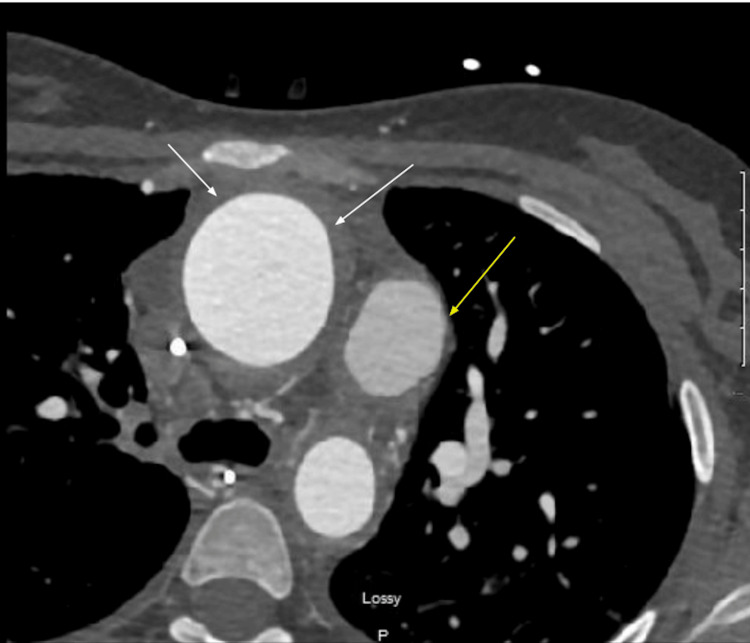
CT angiogram of the chest showing aortic wall thickening The white arrows show the ascending aorta with thickened walls, and the yellow arrow points towards the main pulmonary artery. CT: computed tomography
